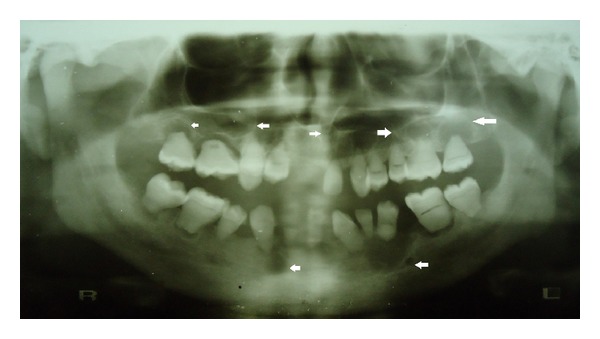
Cropped panoramic radiograph showing generalized short and tapered roots, pulpal obliteration, horizontal/crescent shaped pulpal remnants in pulp chambers, and multiple periapical radiolucencies (white arrows).
